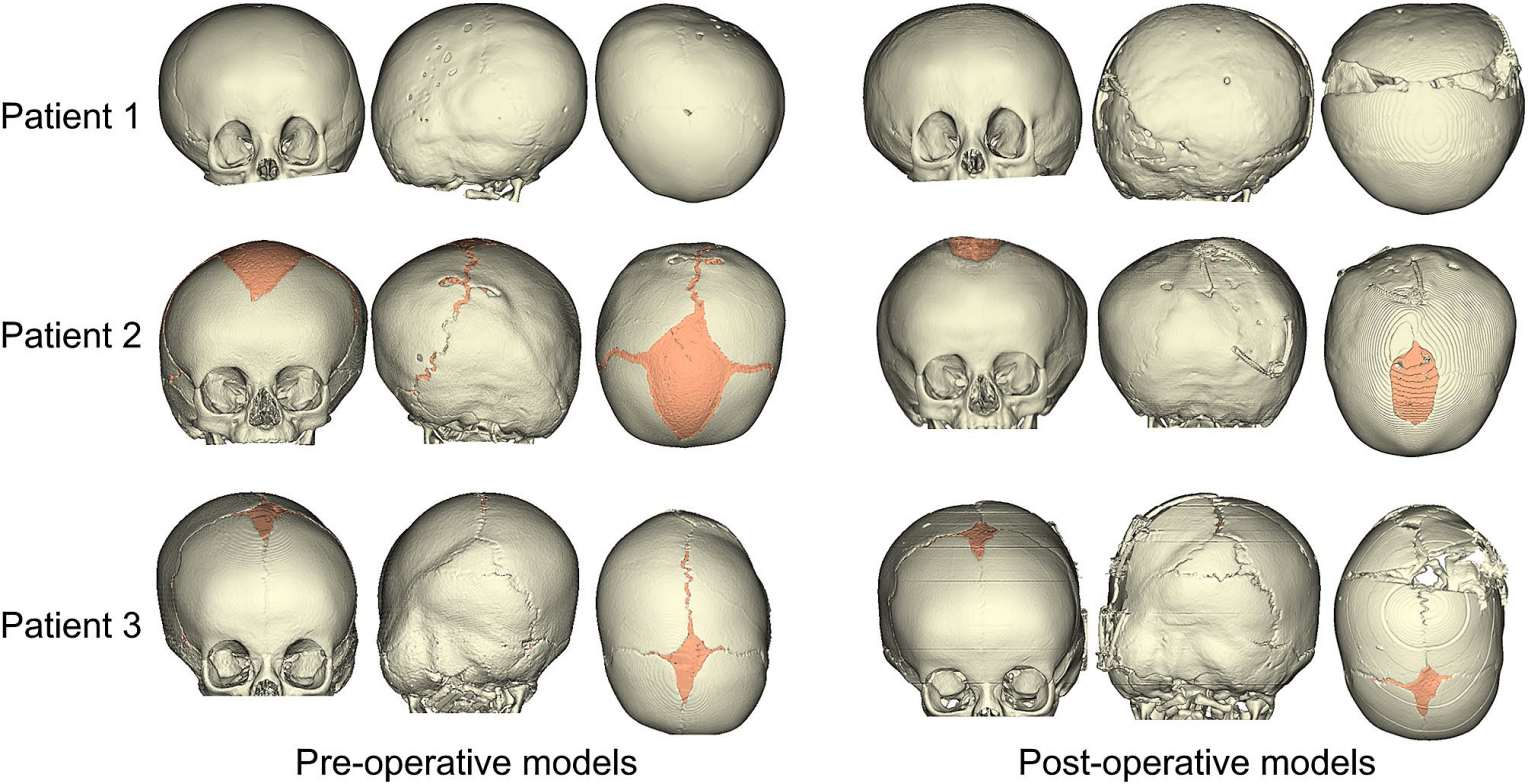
Patient-specific pre and post-operative skull models reconstructed from the CT images.
